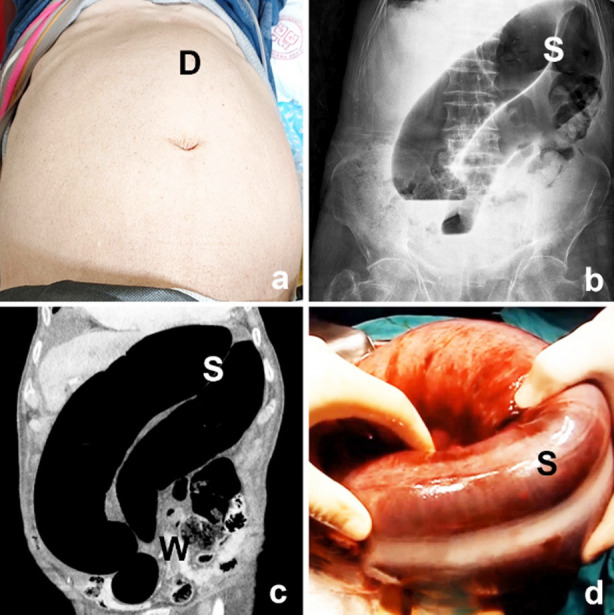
Clinical, radiological, and operative findings in diabetic patients with sigmoid volvulus. a. Clinical appearance (D: left-sided abdominal distention). b. Abdominal x-ray image (S: dolichocolic sigmoid colon). c. Abdominal coronal computed tomography image (S: dolichocolic sigmoid colon, W: whirled sigmoid mesentery). d. Operative appearance (S: dolichocolic sigmoid colon).
